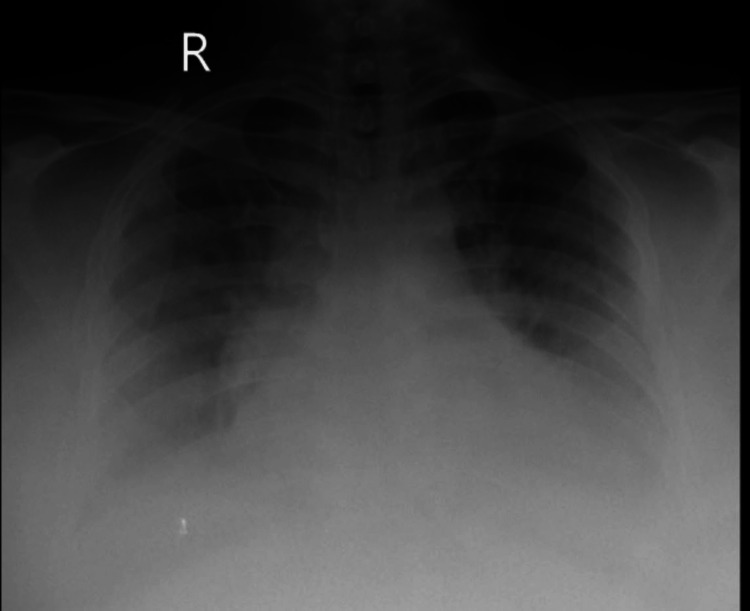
Chest X-ray anteroposterior (AP) view suggestive of bilateral lower-zone haziness
